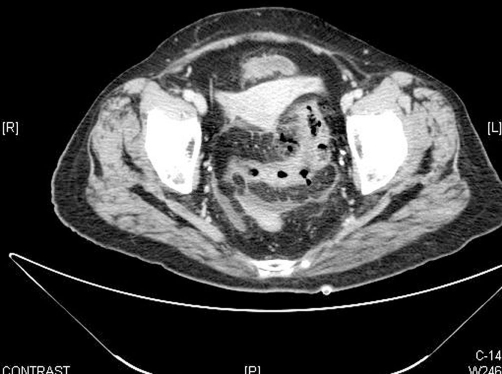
Abdominal CT scan revealed mass and diverticulosis of sigma and perforated cancer with pneumretrooperitoneum.
